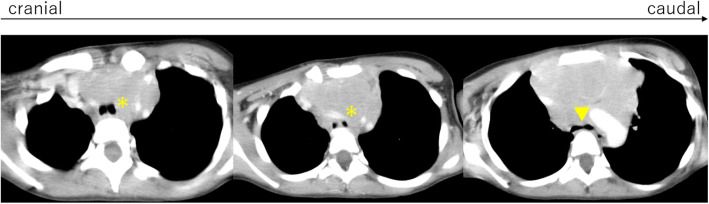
Computed tomography images of a patient who underwent chemotherapy without a biopsy. The trachea was severely compressed by the anterior mediastinal tumor (non-Hodgkin lymphoma). *; tracheal compression by the tumor at the trachea. ▼; tracheal bifurcation
